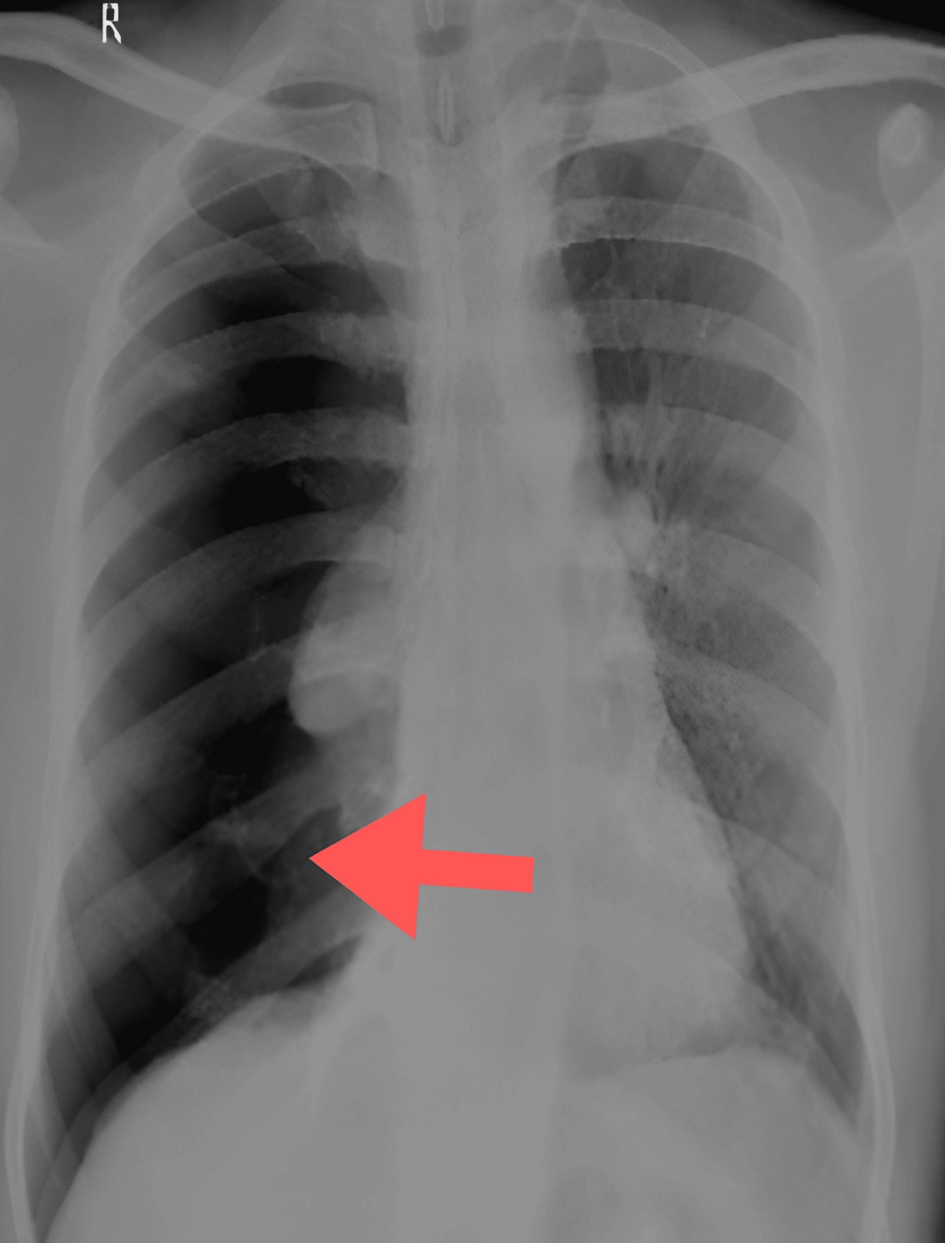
Chest X-ray showing right-sided pneumothorax (arrow).
